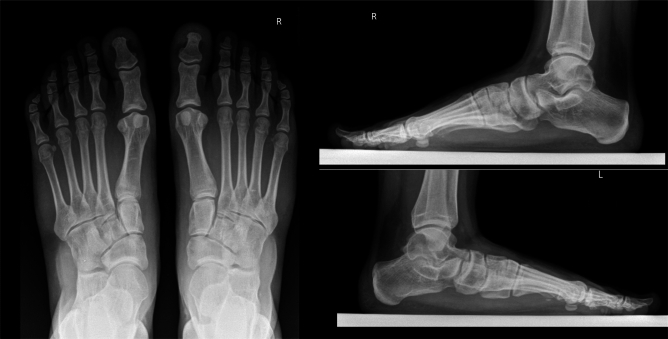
Pre-surgical weight bearing x-ray of right foot of a children affected by pronation-distortion syndrome
